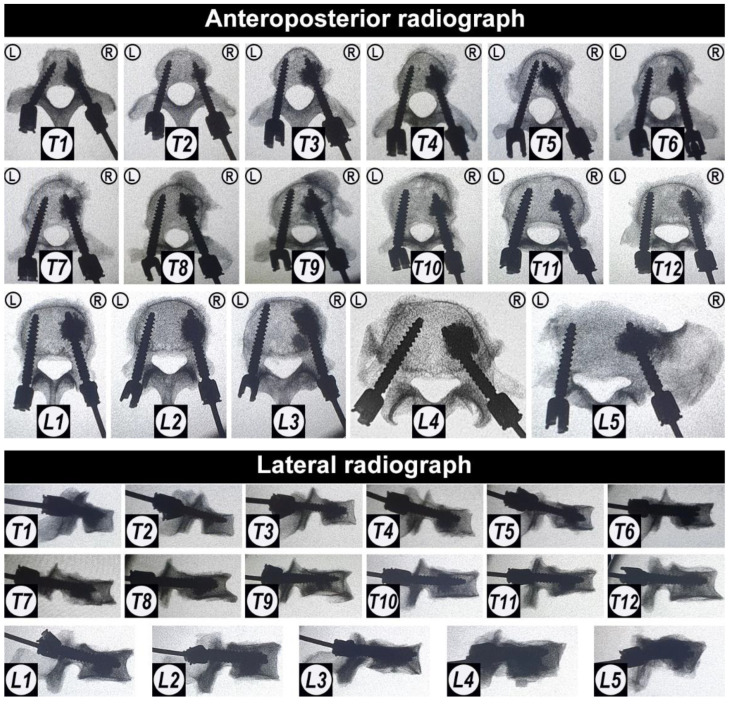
The anteroposterior radiograph and the lateral radiograph of the fenestrated pedicle screws augmented with CaS/HA cement. “L” and “R” indicate the left and right side; T1–T12 and L1–L5 indicate the thoracic and lumbar position of the vertebrae.
